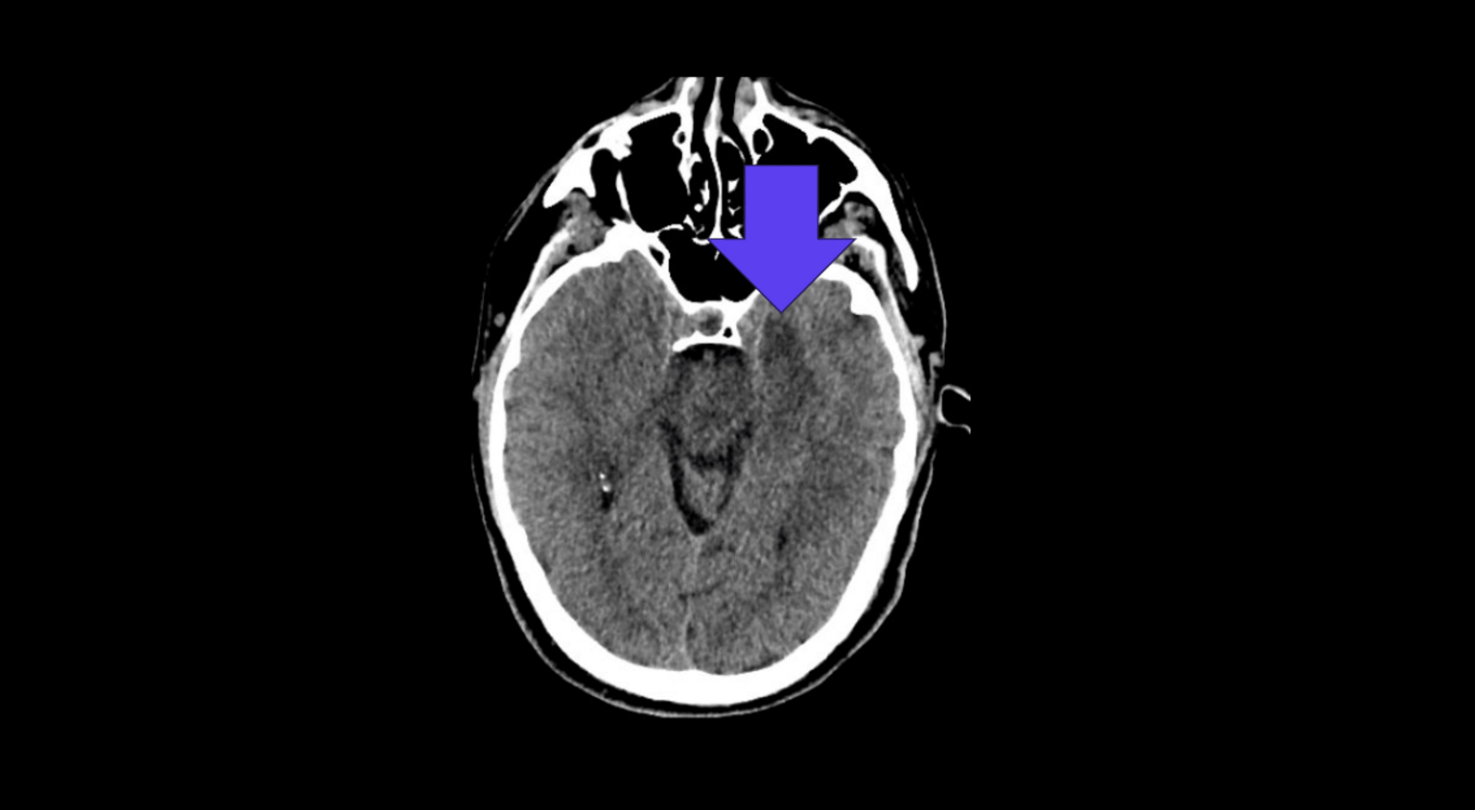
The repeated CT head showing hypodensity of the left medial temporal lobe.
